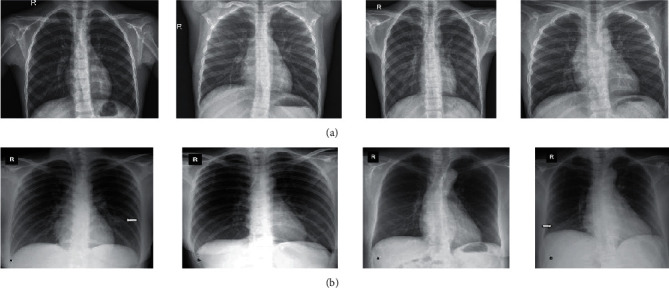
Some examples of the chest X-ray images collected from these datasets: (a) normal case and (b) COVID-19 case.
